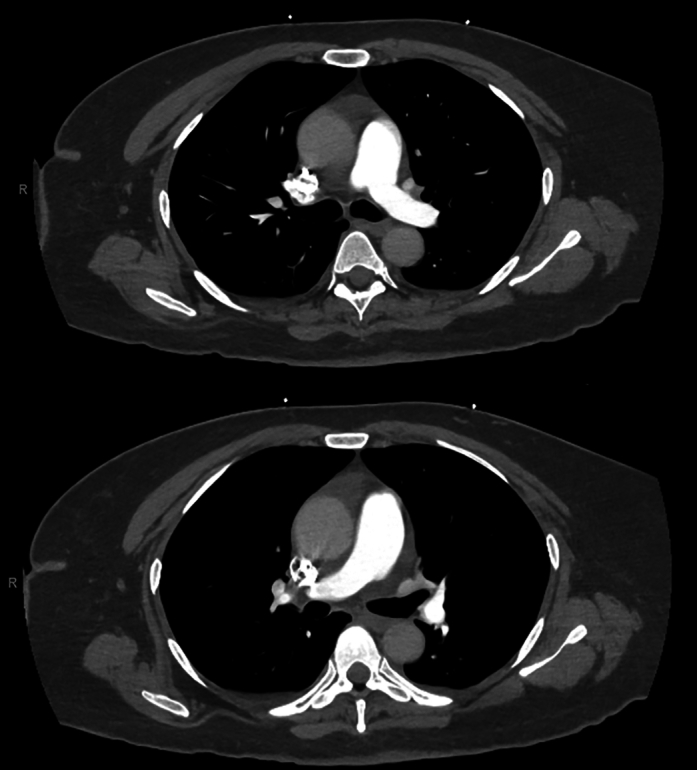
Computed Tomography Angiography of the Chest Axial computed tomography angiography image obtained on admission, demonstrating no evidence of pulmonary embolism.
